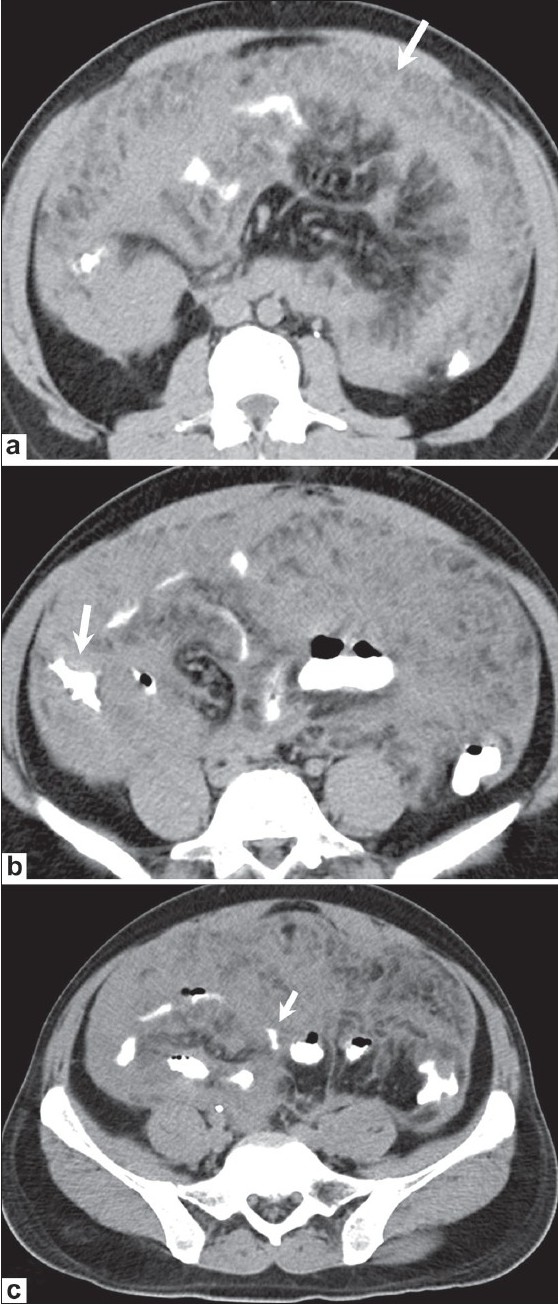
Lymphoma. A 36-year-old male presented with abdominal pain. Axial, contrast-enhanced CT scans of the abdomen show diffuse omental thickening (arrow in a) with bowel wall thickening in the ascending colon (arrow in b) and small bowel (arrow in c). Biopsy was consistent with small-cell, cleaved non-Hodgkin lymphoma
